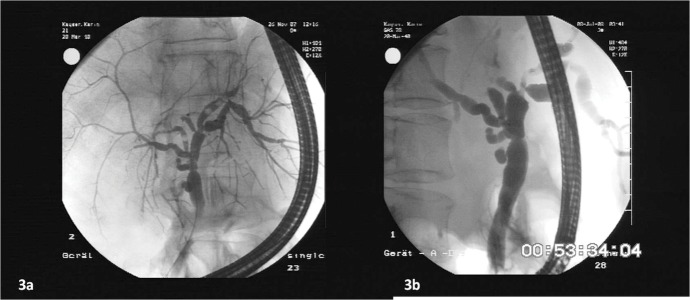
Figure 3a and Figure 3b Demonstrate a Diffuse ITBL Without Bile Duct Dilatations on ERCP. The Diagnosis Was Missed Completely by Ultrasound
